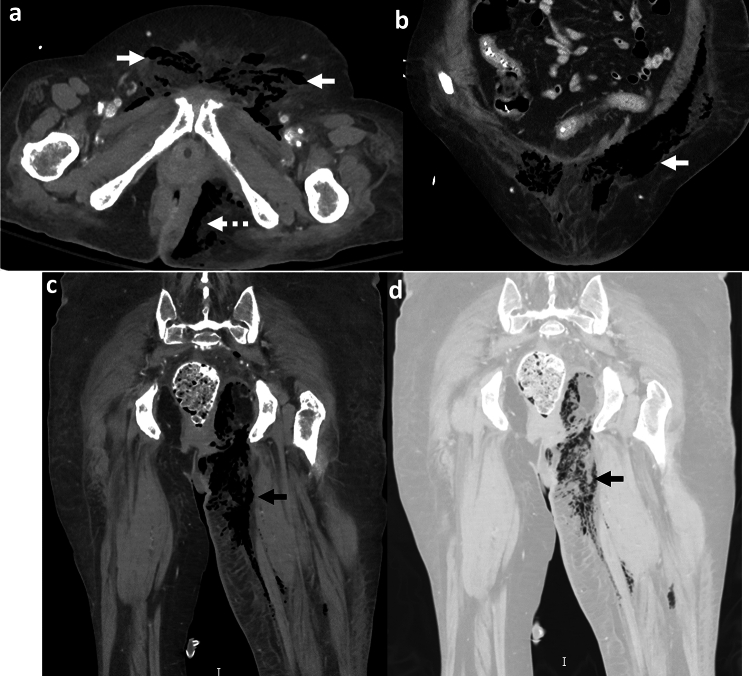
Necrotizing fasciitis of abdominal wall. A 77-year-old with diabetes, dysuria, fever, and pain. Clinical exam showed edema and erythema in the perineal region and left thigh with skin necrosis. CT images show subcutaneous air with fat stranding and fascial thickening in the anterior abdominal wall (solid white arrows, a,b), left gluteal region (dotted white arrow, a), and left upper thigh (black arrow, c,d). Surgical debridement was performed. Cultures showed admixed *Clostridium*, *Bacteroides, Streptococcus*, *Staphylococcus* species, and anaerobes. The patient expired because of fulminant sepsis
